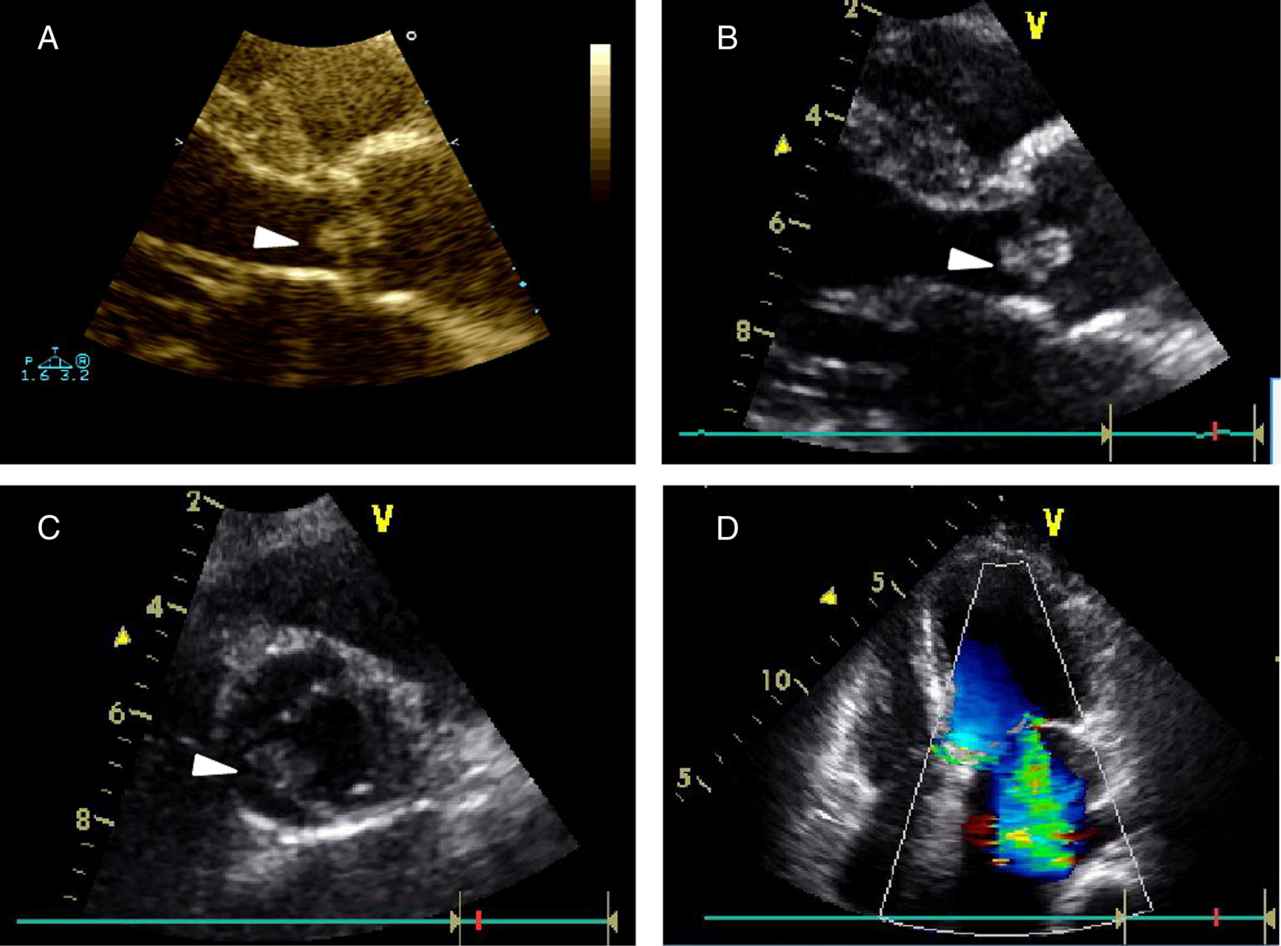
Transthoracic echocardiography. a, parasternal long-axis view shows a round mass (6 × 5 mm, arrow) on the aortic side of the non-coronary cusp of the aortic valve in 2010. b, parasternal long-axis view shows a round mass (6 × 5 mm, arrow) on the aortic side of the non-coronary cusp of the aortic valve in 2015. c, parasternal short-axis view shows a round mass (arrow) on the aortic side of the non-coronary cusp of the aortic valve in 2015. d, Apical 4 chamber view shows moderate mitral regurgitation
